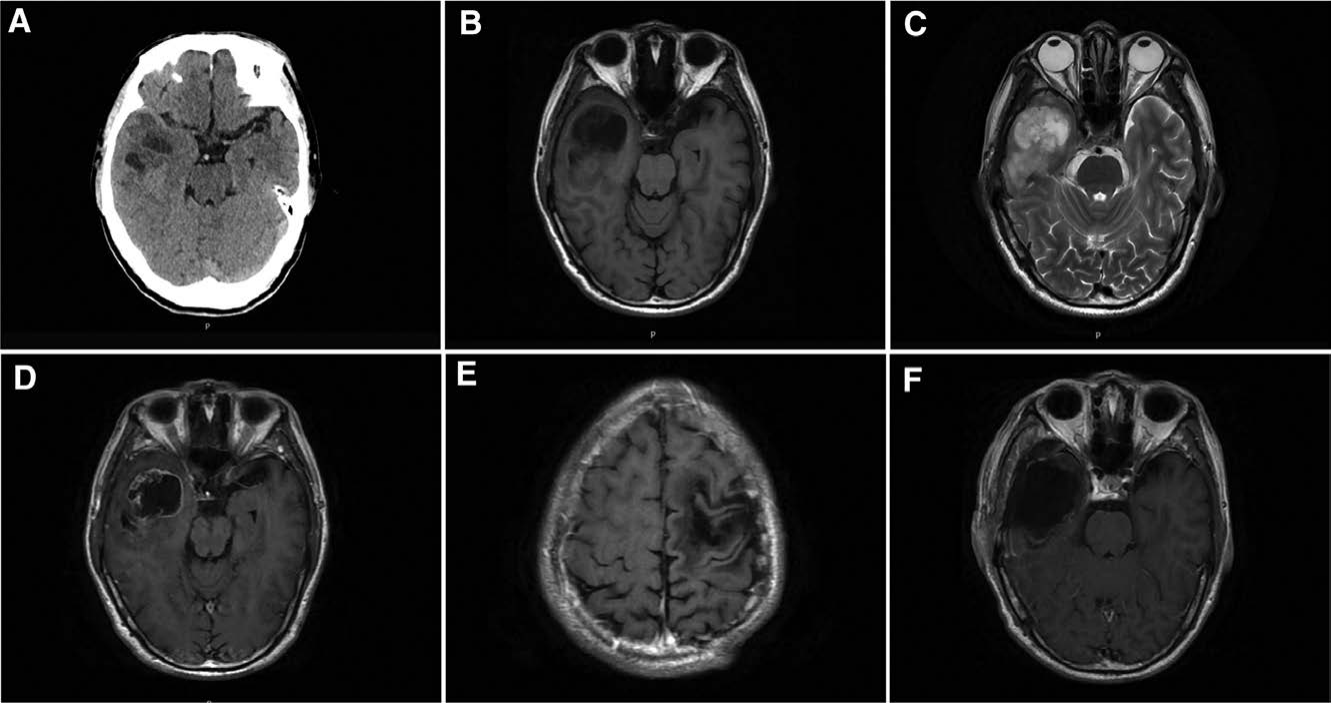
Preoperative CT scans of the GBM (a,7/13/2022); Preoperative MRI scans of the GBM: T1-weighted, T2-weighted and T1-weighted with contrast enhancement (b, c and d, 7/8/2022); Preoperative MRI scans of the meningioma location showed no recurrence: T1-weighted with contrast enhancement (e,7/8/2022); Postoperative MRI scans of the GBM: T1-weighted with contrast enhancement (f, 7/17/2022), CT = computerized tomography, GBM = glioblastoma, MRI = magnetic resonance imaging.
